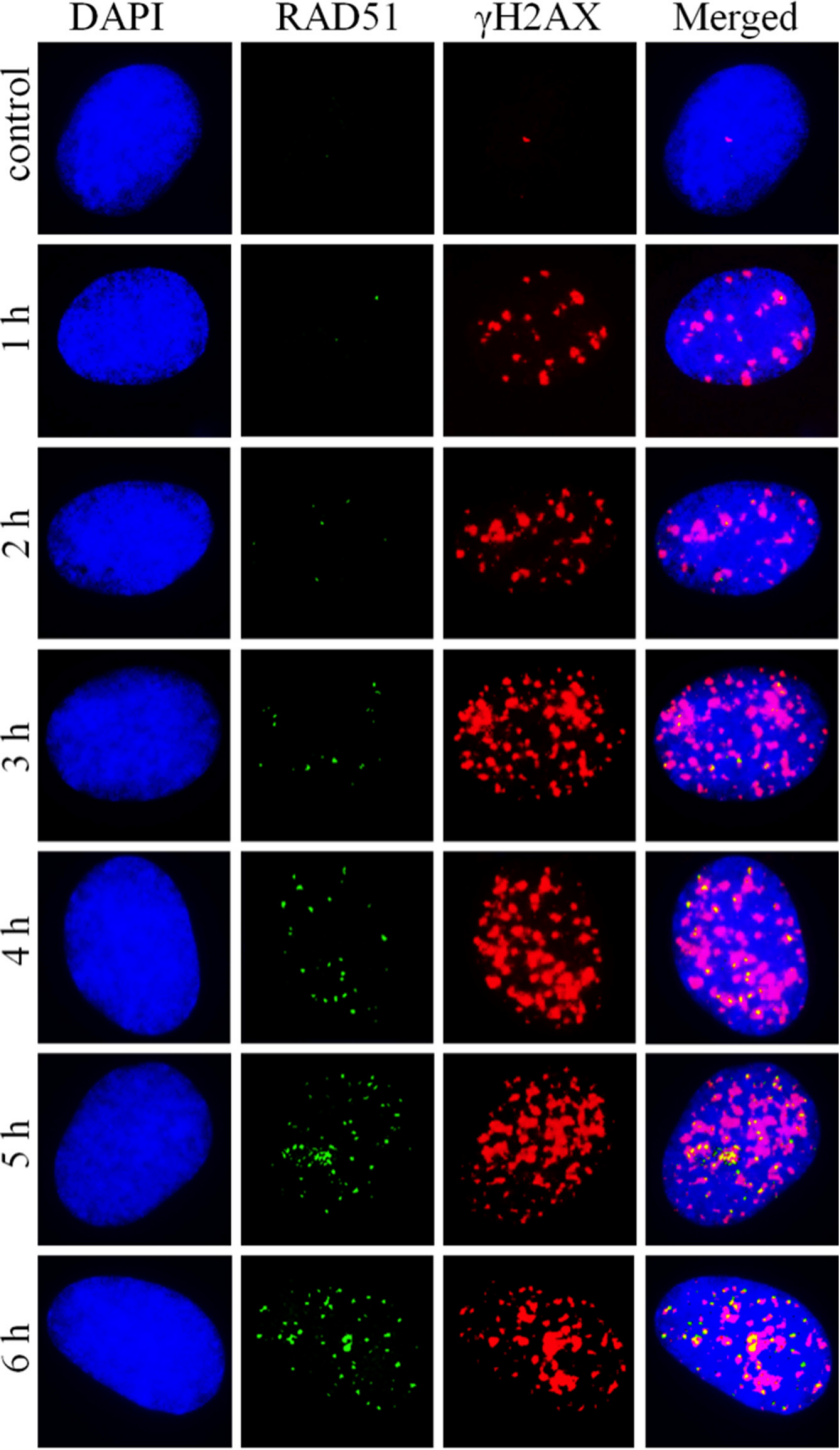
Representative microphotographs of RAD51 and γH2AX foci formed in diploid normal human fibroblasts upon exposure to X-ray radiation at a dose-rate of 4.5 mGy/min
